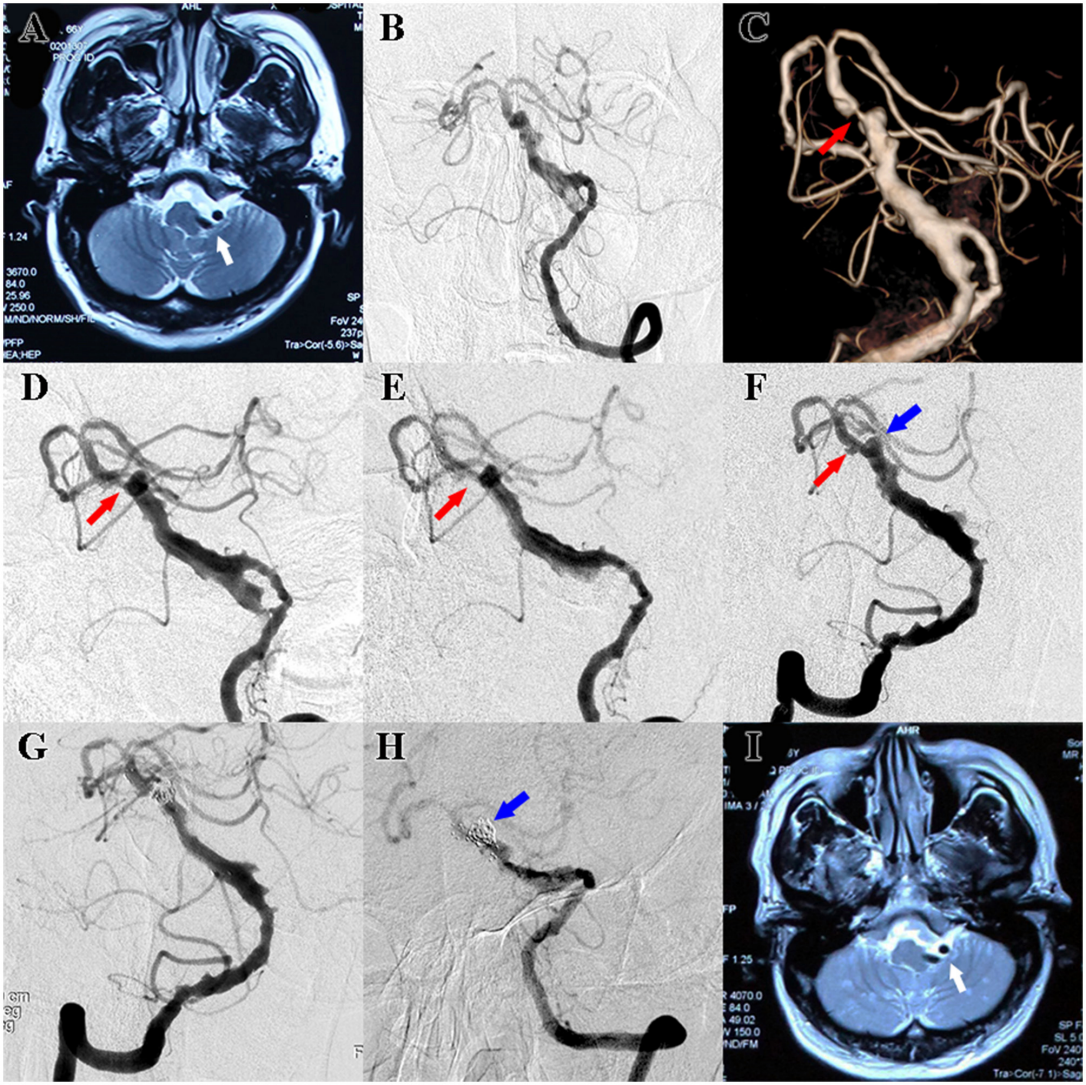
(A) Preoperative T2-weighted MRI showed facial nerve compression resulting from VBD (white arrow). (B) Standard frontal view of preoperative DSA also revealed ectasia, elongation, and tortuosity of the vertebrobasilar artery, and the diagnosis of VBD was confirmed. (C) 3D volume rending reconstructed image of preoperative DSA showed severe stenosis of the basilar artery (red arrow). (D) 2D angiography of optimal view of preoperative DSA demonstrated stenosis of the basilar artery, but the degree of stenosis was less severe (red arrow). (E) Postoperative angiography after balloon angioplasty along with stent implantation showed that the stenosis was partly improved (red arrow). (F) 6-month follow-up angiography showed that the stenosis of the basilar was improved (red arrow) while the aneurysm seemed enlarged (blue arrow). (G) Postoperative angiography after stent-assisted coils embolization showed that the aneurysm was occluded (blue arrow). (H) Follow-up angiography after stent-assisted coils embolization showed that an aneurysm-like protrusion of the VBD was occluded (blue arrow). (I) Follow-up MRI indicated facial nerve compression remained (white arrow).
